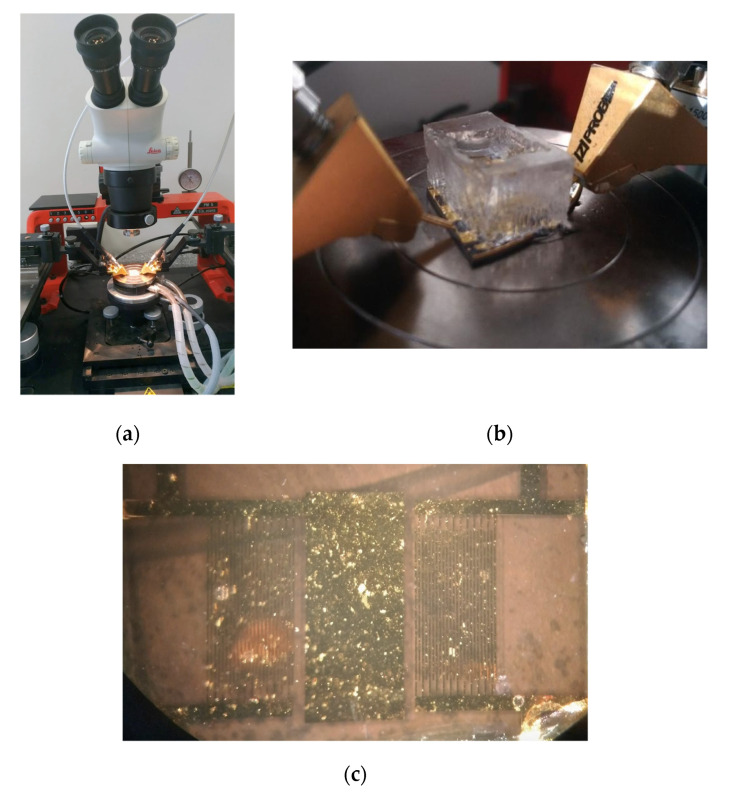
(a) The characterization system consisted of the probe station PM5 SÜSS MicroTec with holder temperature control, two rf-probes (Z-Probe P004 Cascade Microtech Inc., Cheshire, CT, USA), 50 ohm coaxial cables, and an Agilent-5230A vector network analyzer. (b) A fabricated sensor in the probe station during the characterization of glycerol-water solutions. The Z-probes are in contact with the IDT contact pads of the sensor. (c) A device fabricated during an experiment with cell culture media in the fluidic chamber.
